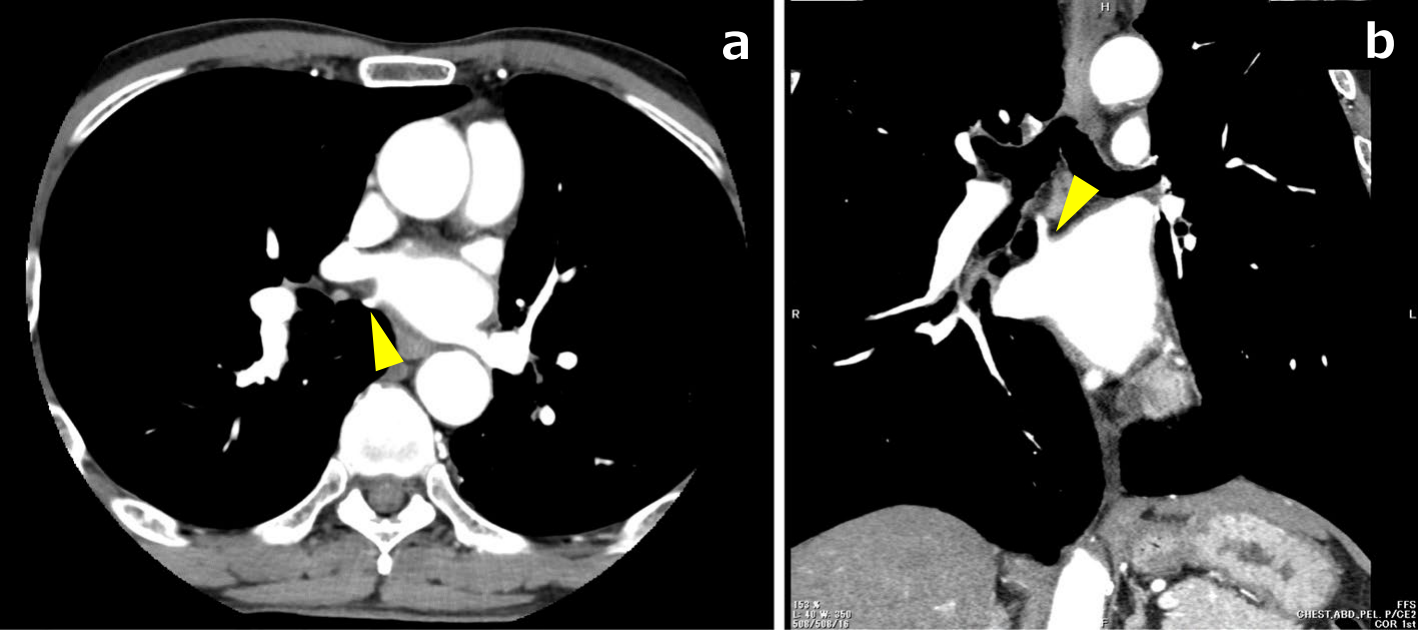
a Preoperative contrast-enhanced computed tomography showing the right top pulmonary vein dorsal to the right main bronchus (yellow arrowhead). b This abnormal vessel merges with the left atrium (yellow arrowhead)
